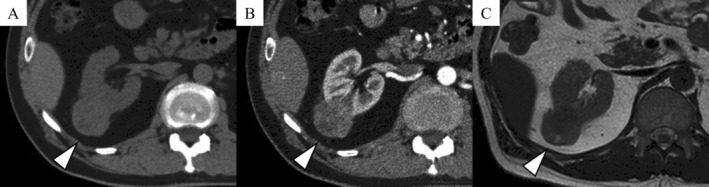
Computed tomography (A: Non‐contrast; B: Contrast‐enhanced) and magnetic resonance imaging (C: T2‐weighted imaging) reveal a 35‐mm tumor in the right kidney (arrows).
